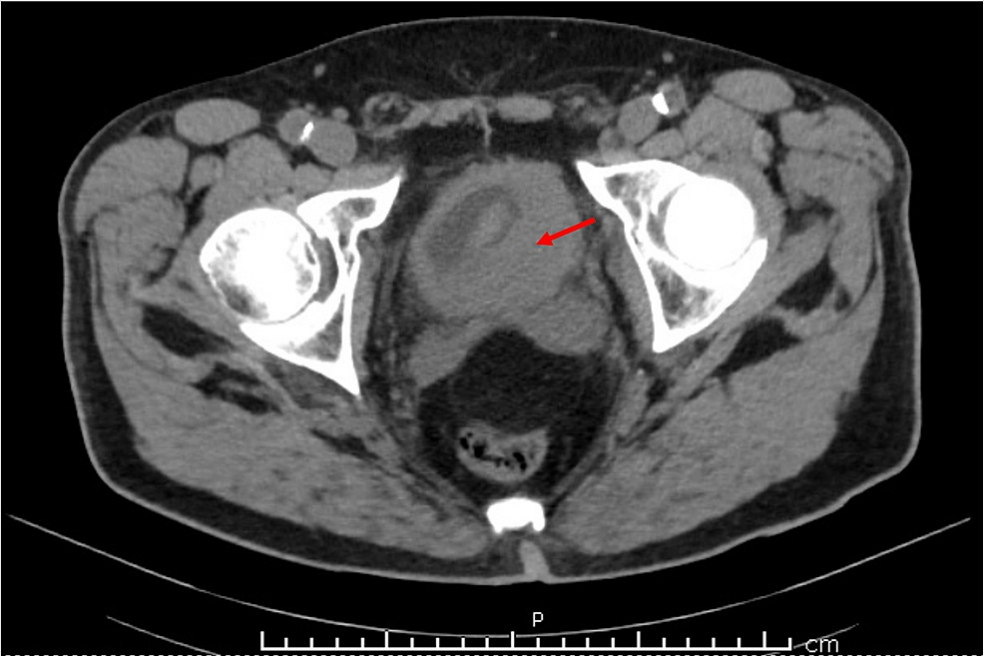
Transverse CT Findings This non-contrast CT scan from a transverse perspective reveals a tumor occupying a large portion of the bladder (highlighted by red arrow). Left-sided lymphadenopathy and vesicular fat infiltration are also visualized.
